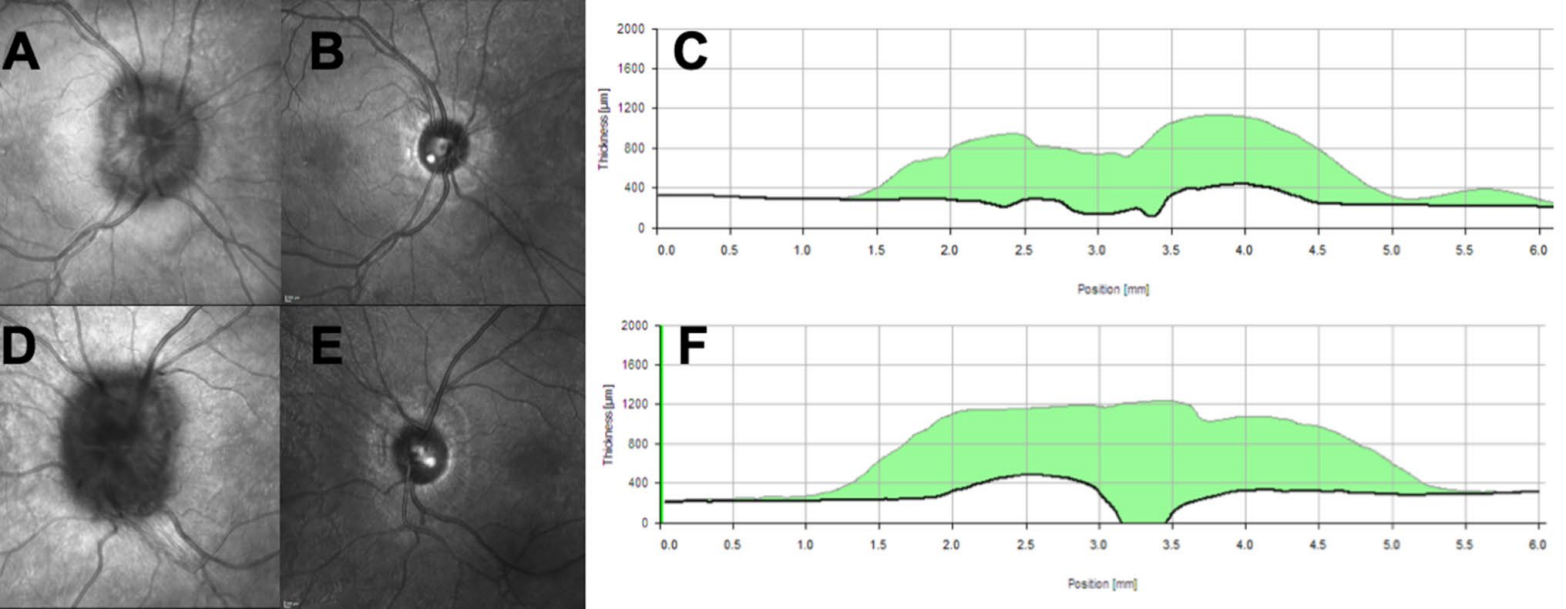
Optical coherence tomography (OCT) images from a young Caucasian woman who presented with papilloedema. CT head and CT venogram showed no abnormalities. CSF opening pressure was 52 cm CSF, with normal contents. Her weight was 99 kg and body mass index 40.2 kg/m^2^. Following lifestyle intervention of a calorie-controlled diet and exercise alone, she lost 15 kg (BMI 34.1) putting her disease into complete remission within 8 weeks. a Infrared image of the right optic nerve; b infrared image of the right optic nerve following weight loss; c shows the central cross section, and the amount of reduction in the retinal nerve fibre layer in the right eye over the 8 weeks. d Infrared image of the left optic nerve; e infrared image of the left optic nerve following weight loss; f shows the central cross section and the amount of reduction in the retinal nerve fibre layer in the left eye over the 8 weeks
